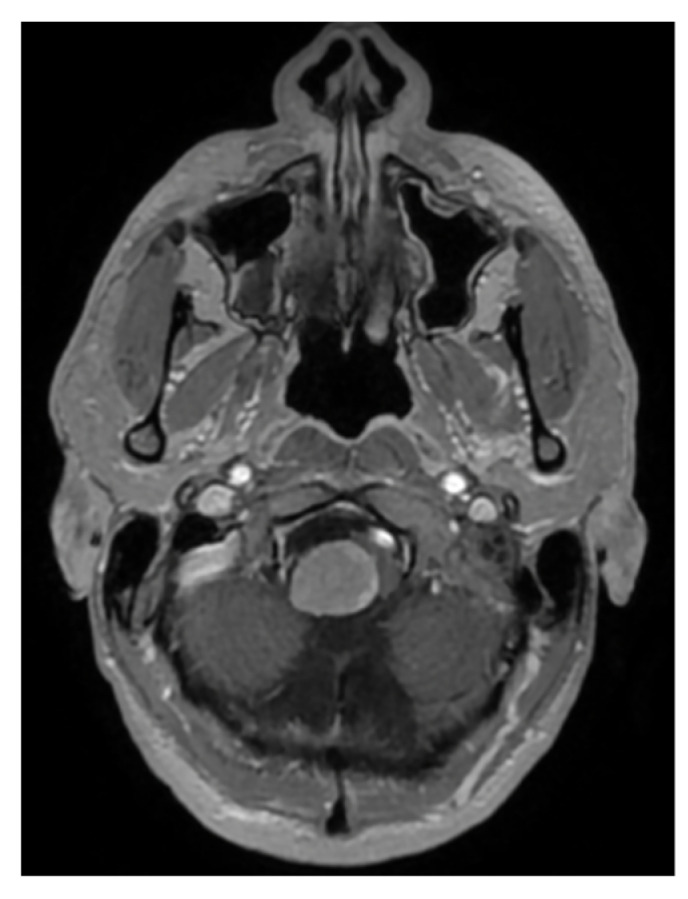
Single cross-sectional scan of a right-sided foramen magnum meningioma with mild brainstem modeling. MRI T1 + C.
